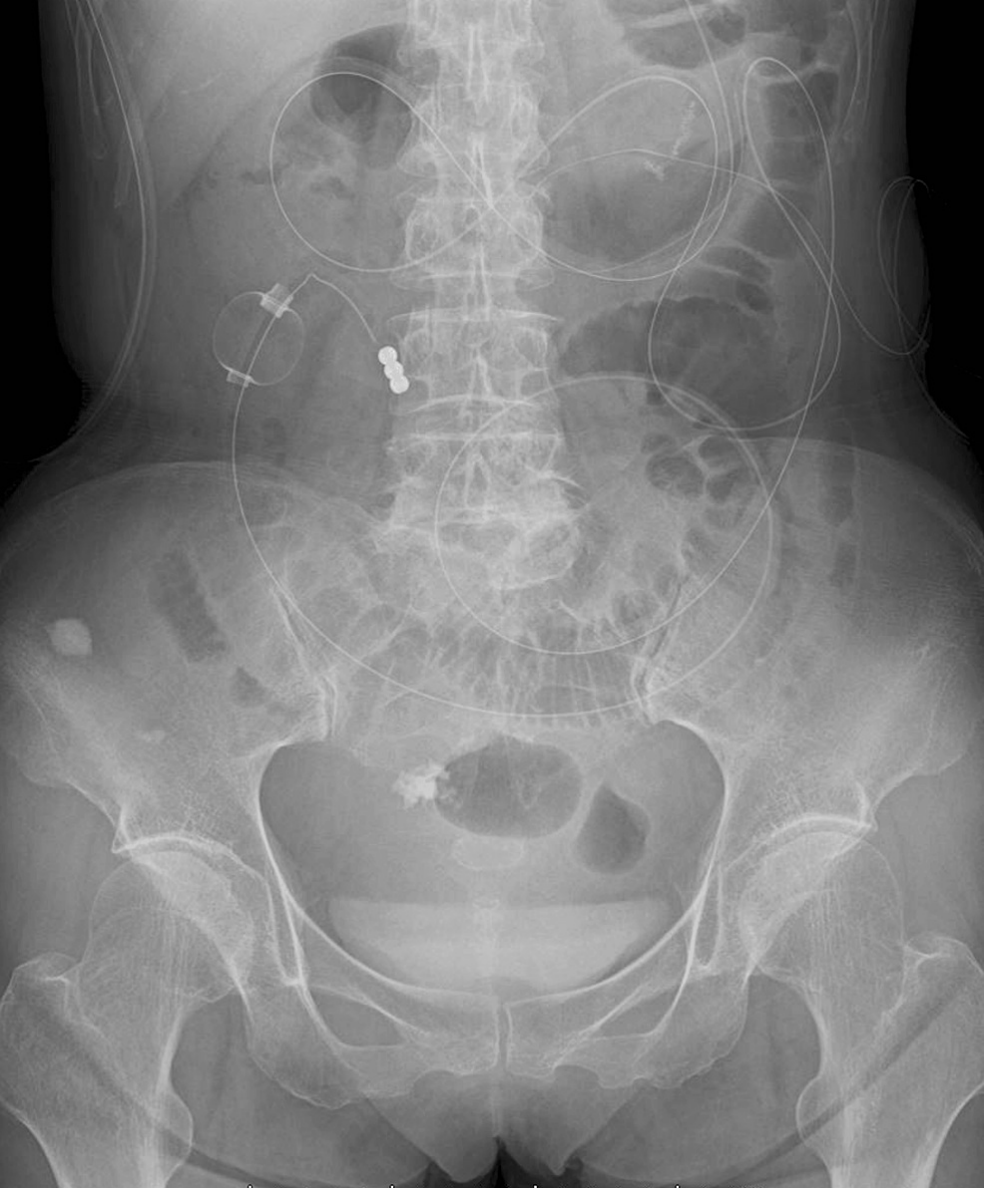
X-ray photograph after intubating a long tube A long tube was intubated into the dilated small bowel.
